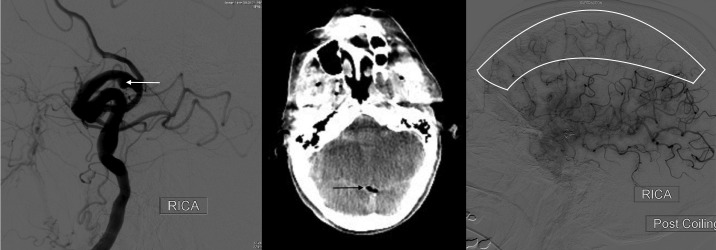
Imaging results. A. Internal carotid artery lateral view computed tomography (CT) angiogram demonstrates a 5 mm x 3 mm blister aneurysm (white arrow) at the origin of the Posterior communicating artery. B. Post-interventional axial CT head demonstrating air (black arrow) in the straight sinus. C. Internal carotid artery lateral view digital subtraction angiogram in the capillary phase demonstrating filling defects (area surrounded by white lines) caused by air embolization in territory of middle cerebral artery.
